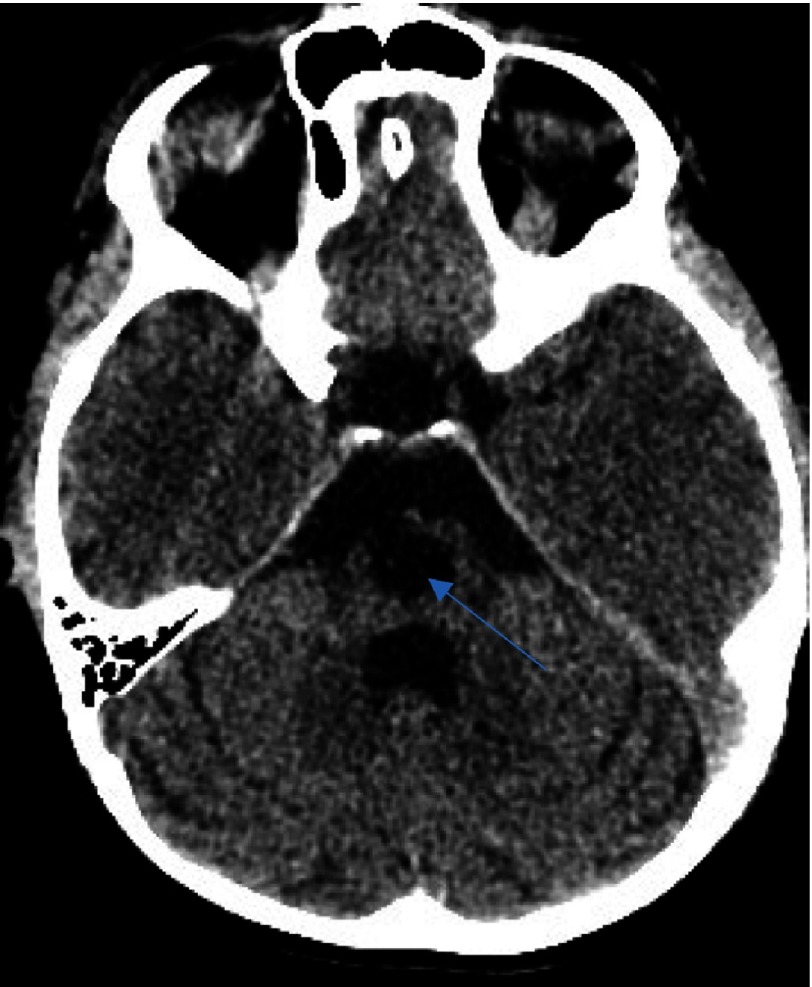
Same CT scan, at a higher level, visualizing infarction at the ponto-mesencephalic junction (arrow).
